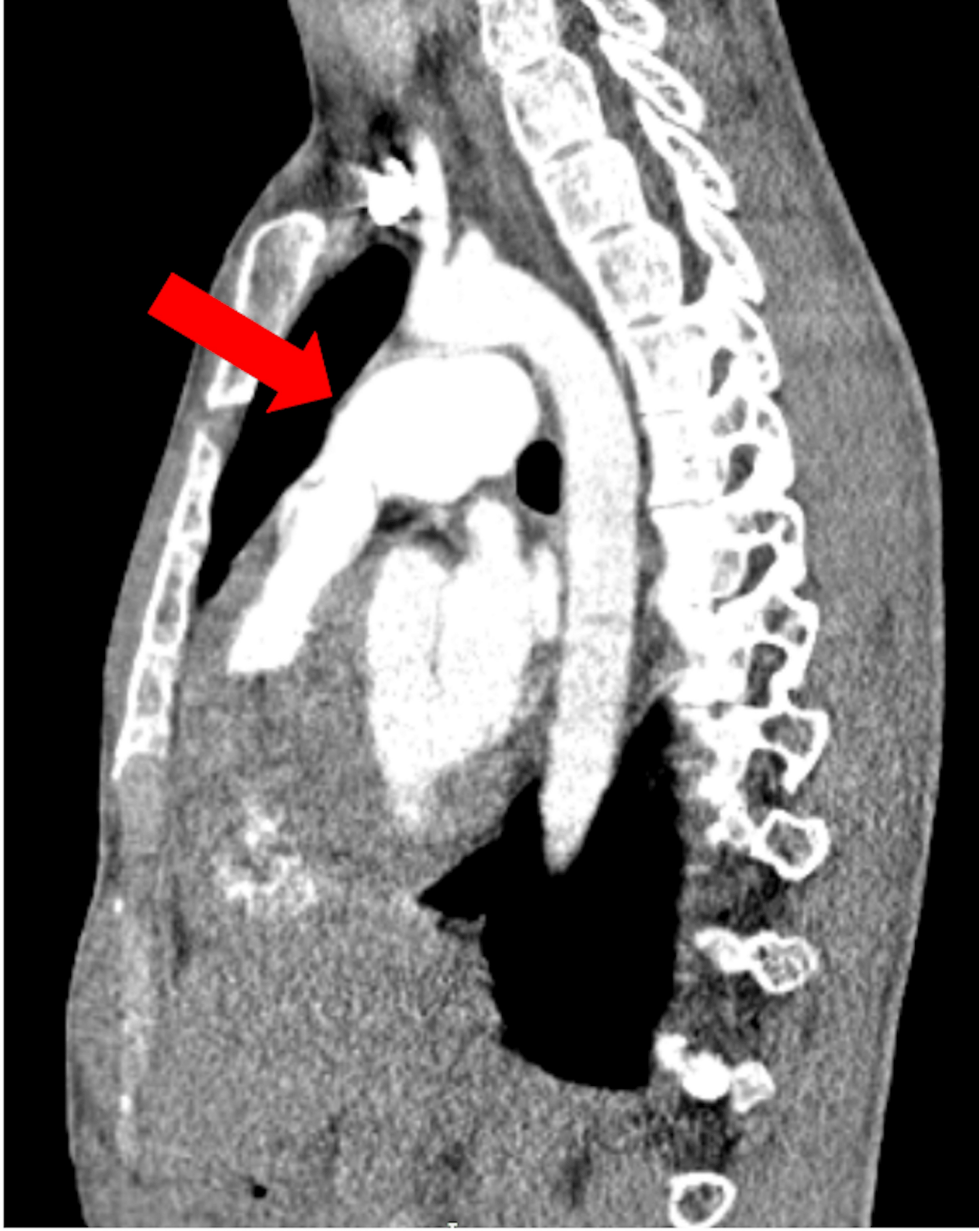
Sagittal CTPA Showing Post-Stenotic Dilatation of the Left Pulmonary Artery Marked dilatation of the left pulmonary artery (red arrow) is seen, consistent with post-stenotic pulmonary artery dilatation secondary to severe pulmonary valve stenosis. No evidence of pulmonary embolism or associated aortic coarctation is observed. CRPA: computed tomography pulmonary angiography
